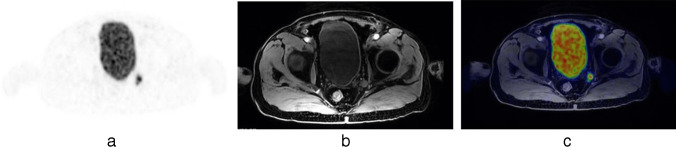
[^68^Ga]Ga-PSMA-11 PET/MRI scan identified a suspicious lymph node at the level of the left obturatory region, as shown in PET (a), MRI (b) and PET/MRI (c) axial view
